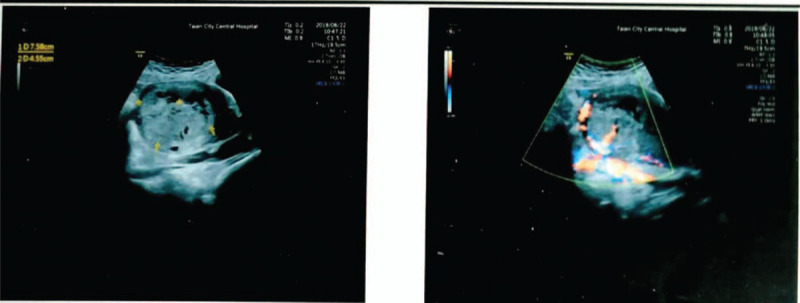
Ultrasound showing an uneven echo group of 7.6 × 4.6 cm in the bottom of the right uterine cavity, which was not clearly demarcated from the posterior wall. The muscle layer became thinner, with a thinnest part of about 0.19 cm. Abundant blood flow signals were observed.
